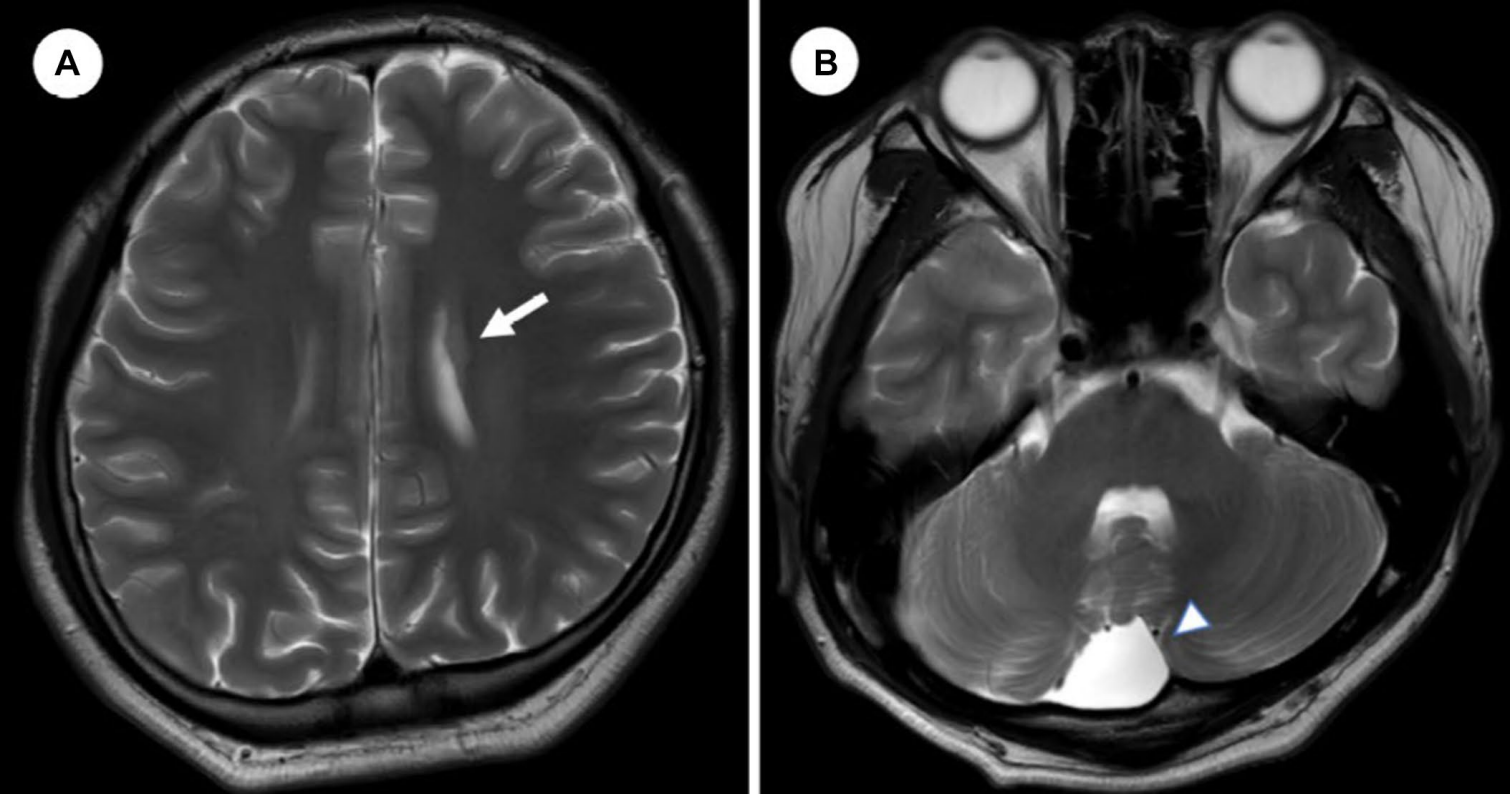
Brain magnetic resonance image. (A) An axial image from T2/FLAIR of brain shows a 1 cm sized gray matter heterotopia in frontal periventricular area. (B) A 4 cm sized arachnoid cyst in retrocerebellar posterior cranial fossa.
